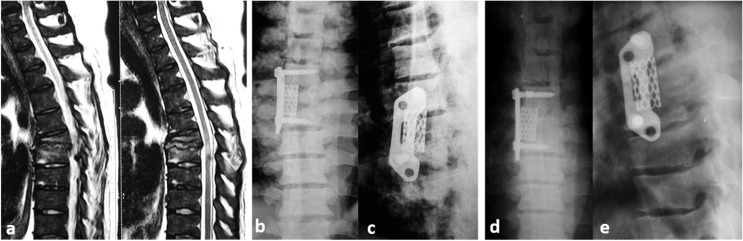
SD D6–7 in a 47-year-old male. (a) Preoperative sagittal MRI veiws. (b and c) Immediate postoperative X-rays showing cropectomy of D6 and fusion D5–7 using titanium cage and plate via anterior approach. (d and e) Follow-up X-rays after 6 months.
